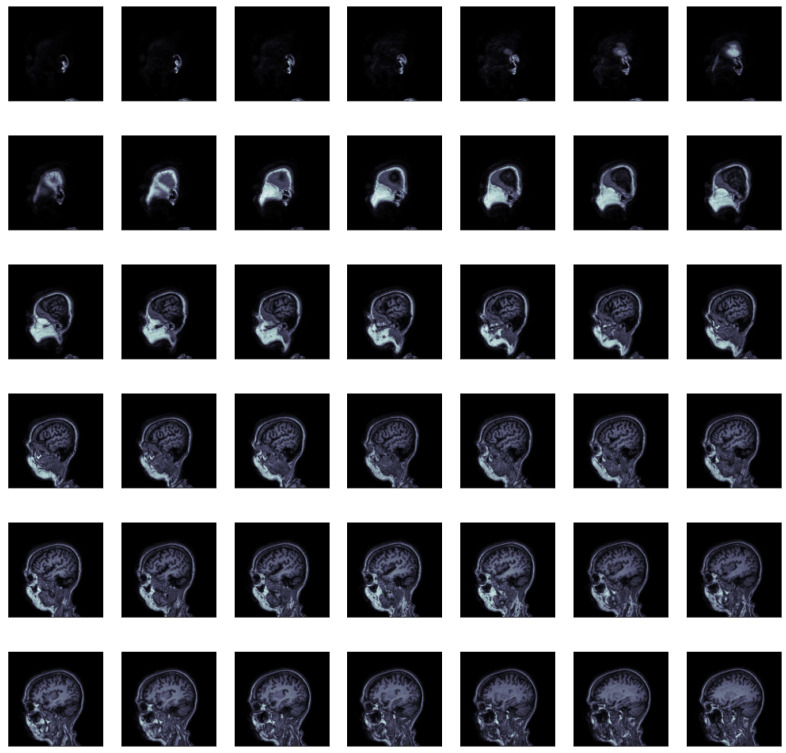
Volumetric data from a subject’s MRI (extracted slice).
